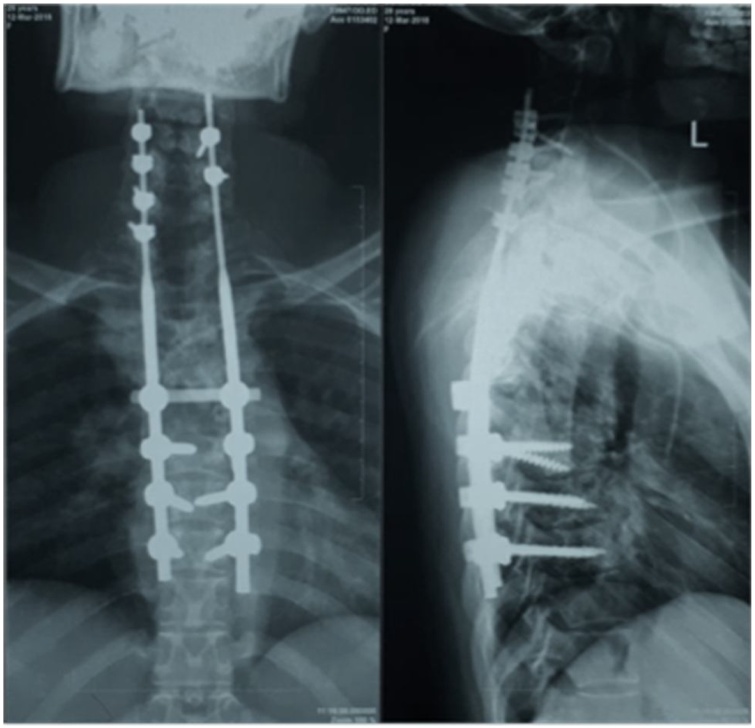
Postoperative radiograph demonstrated pedicle rod and screw construct that resulted in improved kyphotic deformity.
